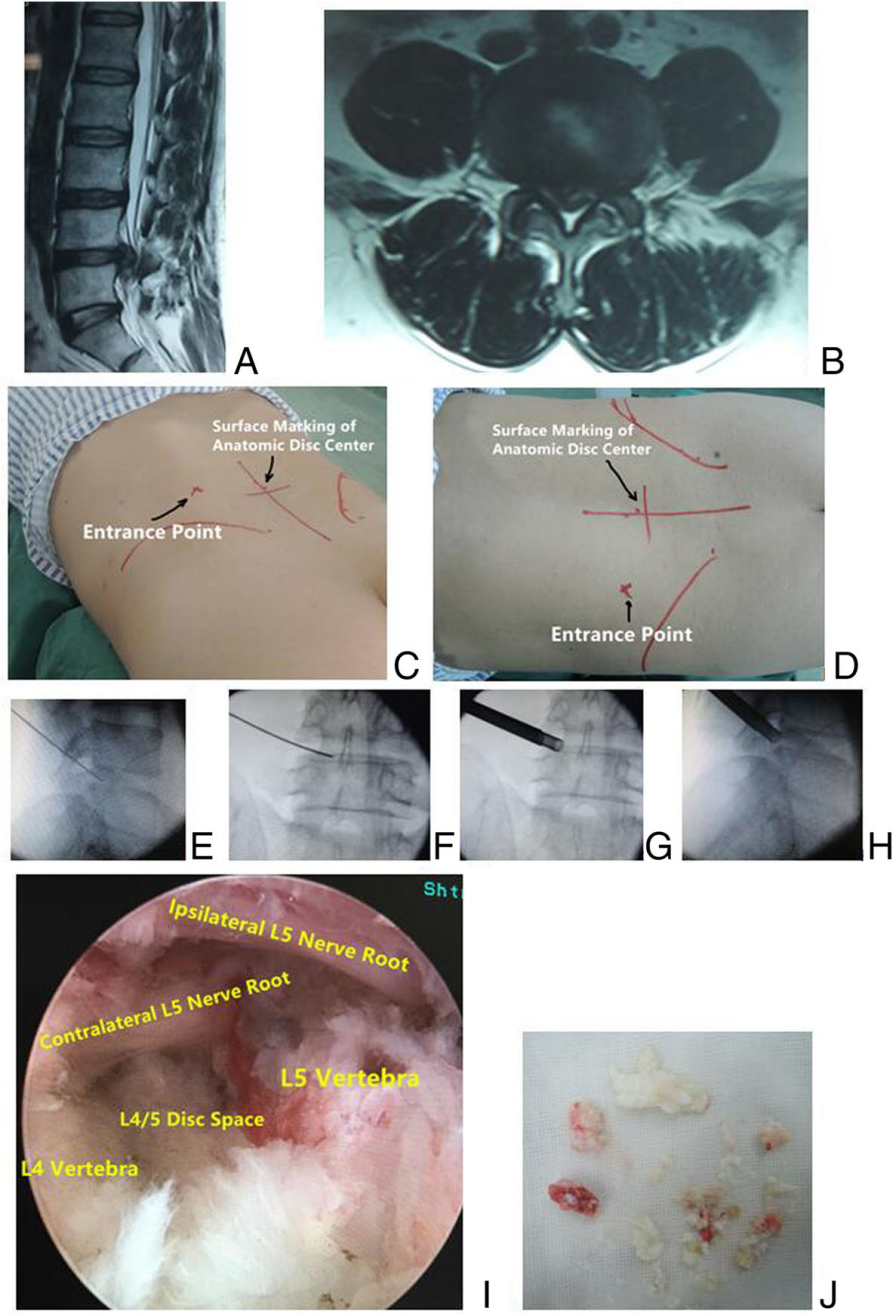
a Sagittal and b axial MR images showed L4/5 disc herniation in 41-year-old man. c, d Photography showed the surface marking of anatomic disc center identified by the intersection of transverse L4/5 level line and longitudinal midline, and the entrance point of puncture located at the corner of the flat back turning to the lateral side. The tip of puncture needle was in the posterior one third of intervertebral space on e lateral C-arm view and beyond the medial border of pedicle on f posteoanterior C-arm view. A 7.5-mm hand reamer was introduced through the cannula to ream away the ventral bone of superior facet joint and ligmentum flavum for enlargement of foramen (press-down enlargement of foramen) until the resistance disappeared. The tip of reamer should exceed the medial border of pedicle till the midline between the pedicle and spinal process on g posteoanterior C-arm view, and it should be close to posterior wall of target disc on h lateral C-arm view. i Endoscopic picture showed that both the ipsilateral nerve root and the contralateral nerve root were exposed for complete decompression after removal of j sequestrated disc fragments
